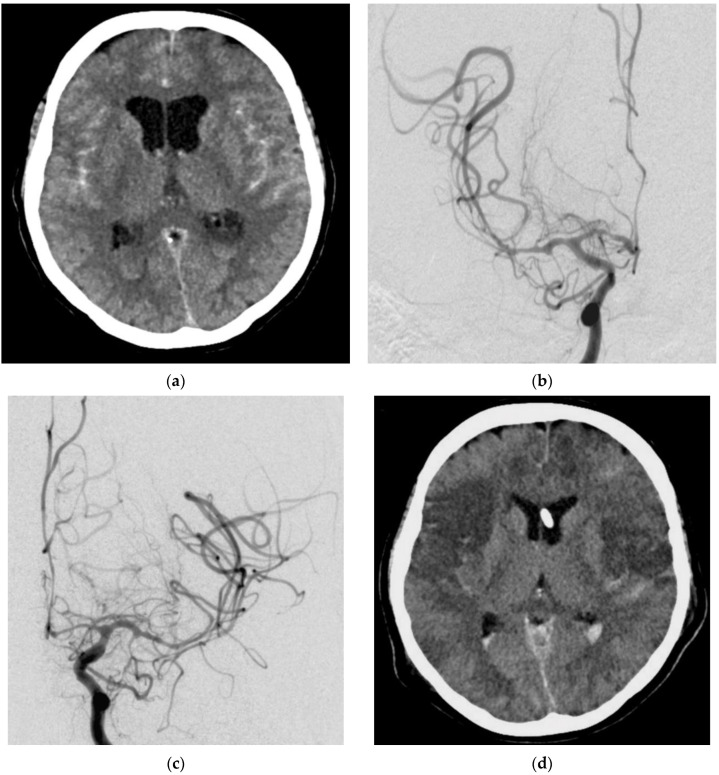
Diagnostic imaging and EVT in a 50-year-old female patient with an aSAH due to a ruptured left ICA aneurysm. Cranial CT (a) showed an aSAH. DSA with contrast-medium injection of both hemispheres revealed massive vasospasm (b,c). Post-interventional CT showed ischemic infarctions of both hemispheres (d).
